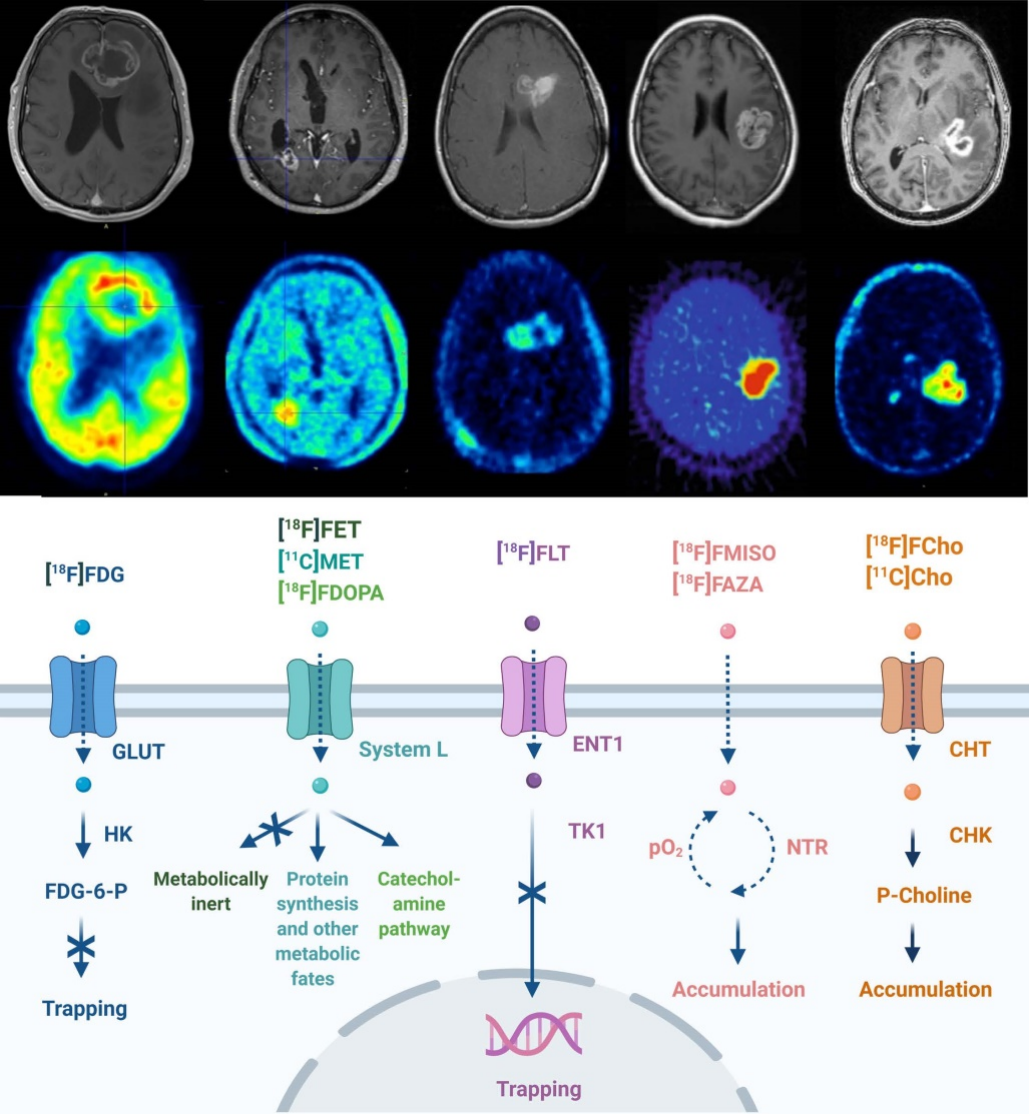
Routine PET imaging in neuro-oncology. PET/CT techniques for neuropathologic imaging are dominated by radiopharmaceuticals focussing on altered glucose metabolism (desoxy-2-[^18^F]fluoro-D-glucose ([^18^F]FDG)), amino acid metabolism (L-[^11^C]-methyl-methionine ([^11^C]MET), O-2-[^18^F]fluoroethyl-L-tyrosine ([^18^F]FET), 3,4-dihydroxy-6-[^18^F]fluoro-L-phenylalanine ([^18^F]F-DOPA)), proliferation (3'-deoxy-3'-[^18^F]fluoro-thymidine [^18^F]FLT)), tumoral hypoxia sensing ([^18^F]fluoro-misonidazole ([^18^F]FMISO), [^18^F]fluoro-azomycin arabinoside ([^18^F]FAZA)), and lipid metabolism ([^11^C]choline ([^11^C]Cho), [^18^F]fluoroethyl-choline ([^18^F]FCho)). Abbreviations: High affinity choline transporter (CHT), choline kinase (CHK), equilibrative nucleoside transporter (ENT), 2'-fluoro-2'-deoxy glucose-6-phosphate (FDG-6-P), glucose transporter (GLUT), hexokinase (HK), nitroreductase (NTR), partial pressure of oxygen (pO_2_), Na+-independent plasma membrane amino acid transport (System L), thymidine kinase (TK). Adapted with permission from 18, copyright 2017 Codon Publications.
